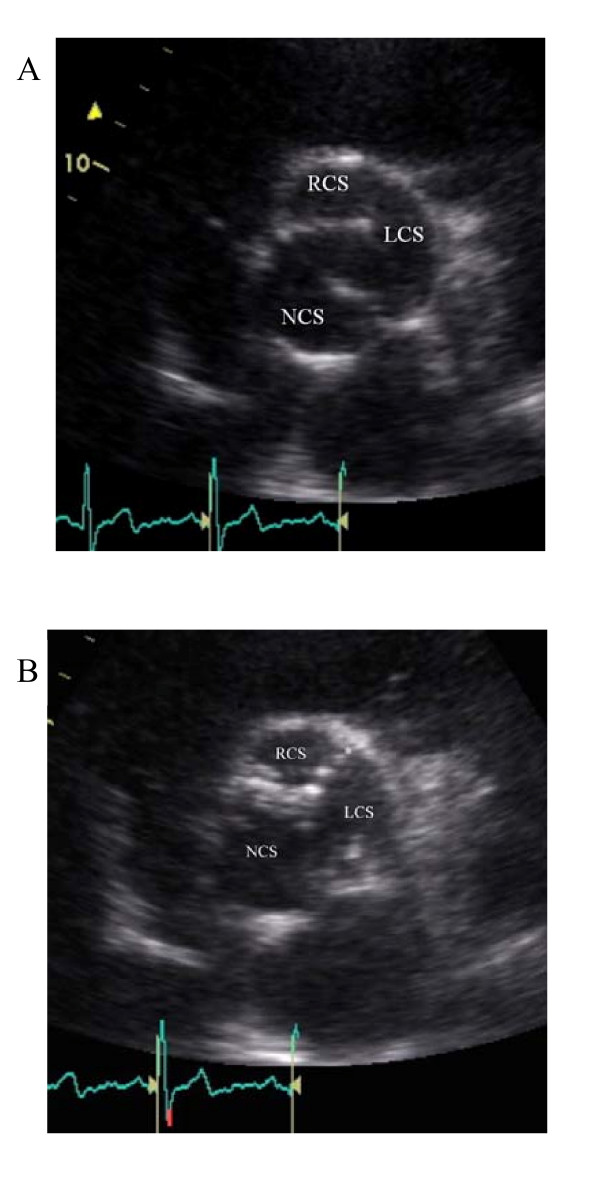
Parasternal short axis view of aortic valve during systole (A) and diastole (B): BAV with "rafe" (*) between the left coronary sinus (LCS) and right coronary sinus (RCS). NCS: non coronary sinus.
